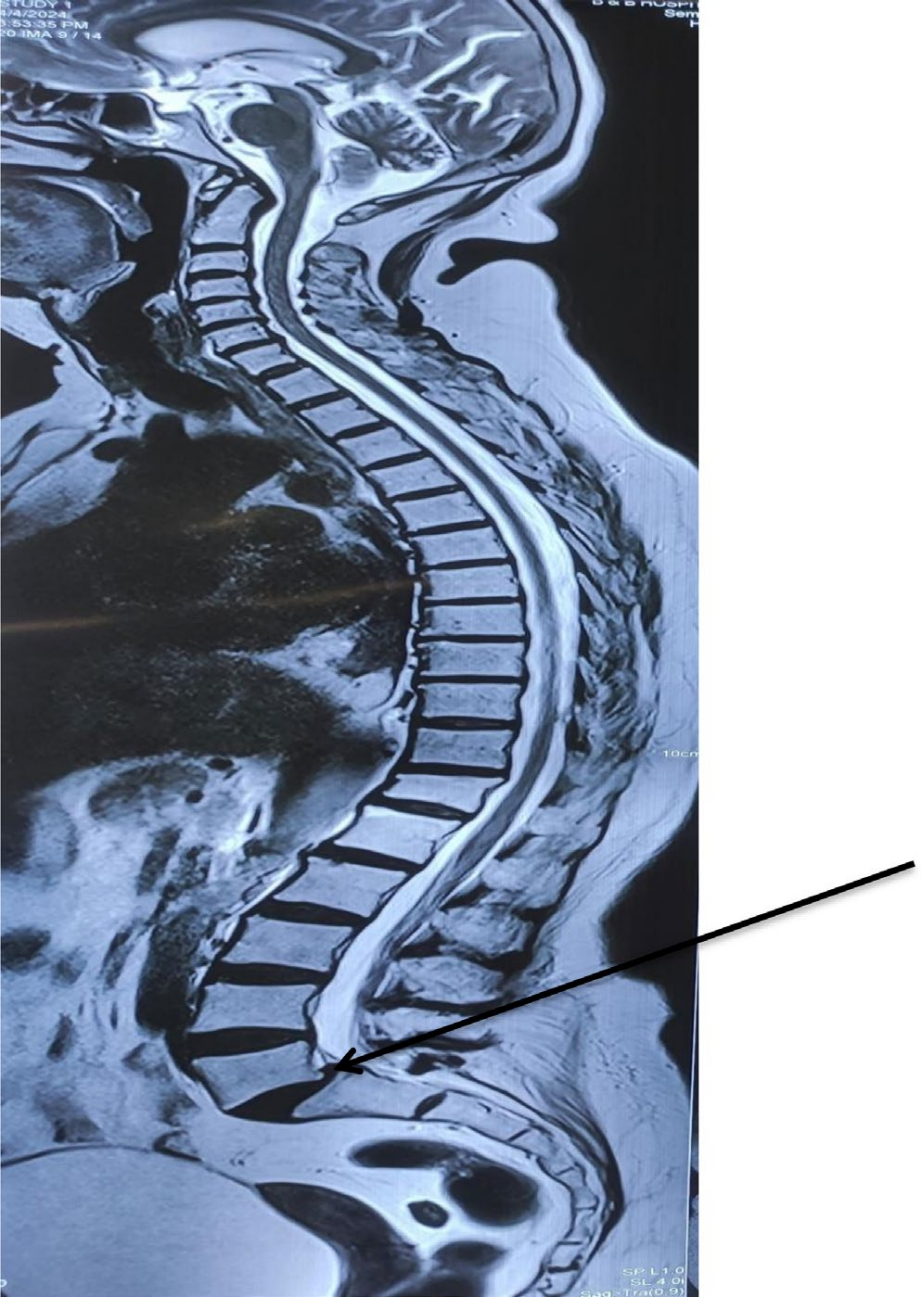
MRI whole spine screening shows features suggestive of posterior subluxation of L5‐S1 joint (black arrow) without any obvious spinal cord or nerve root compression.
